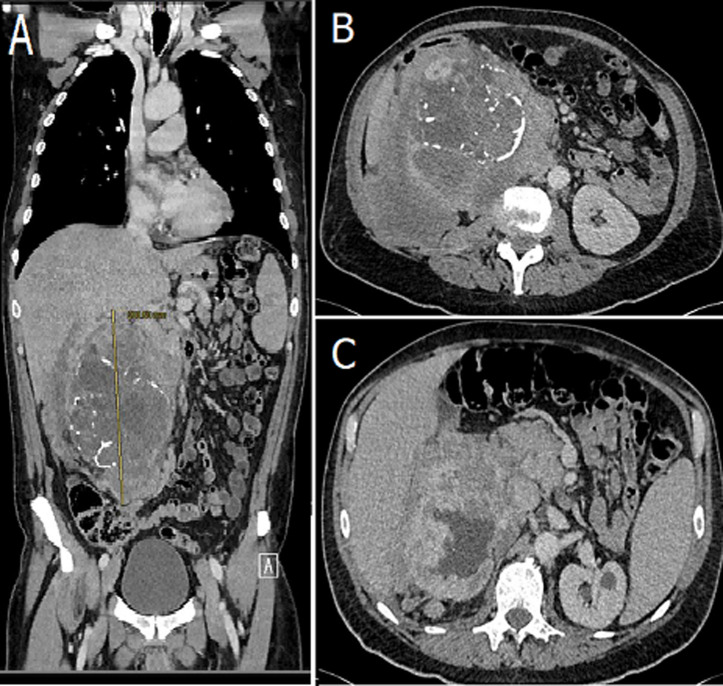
A) abdomen CT scan revealed a retroperitoneal mass of 20cm; B) abdomen CT scan revealed a retroperitoneal mass of 20cm with calcification involving the liver; C) abdomen CT scan revealed a retroperitoneal mass of 20cm with calcification involving the liver, the kidney and surrounding the vena cava
